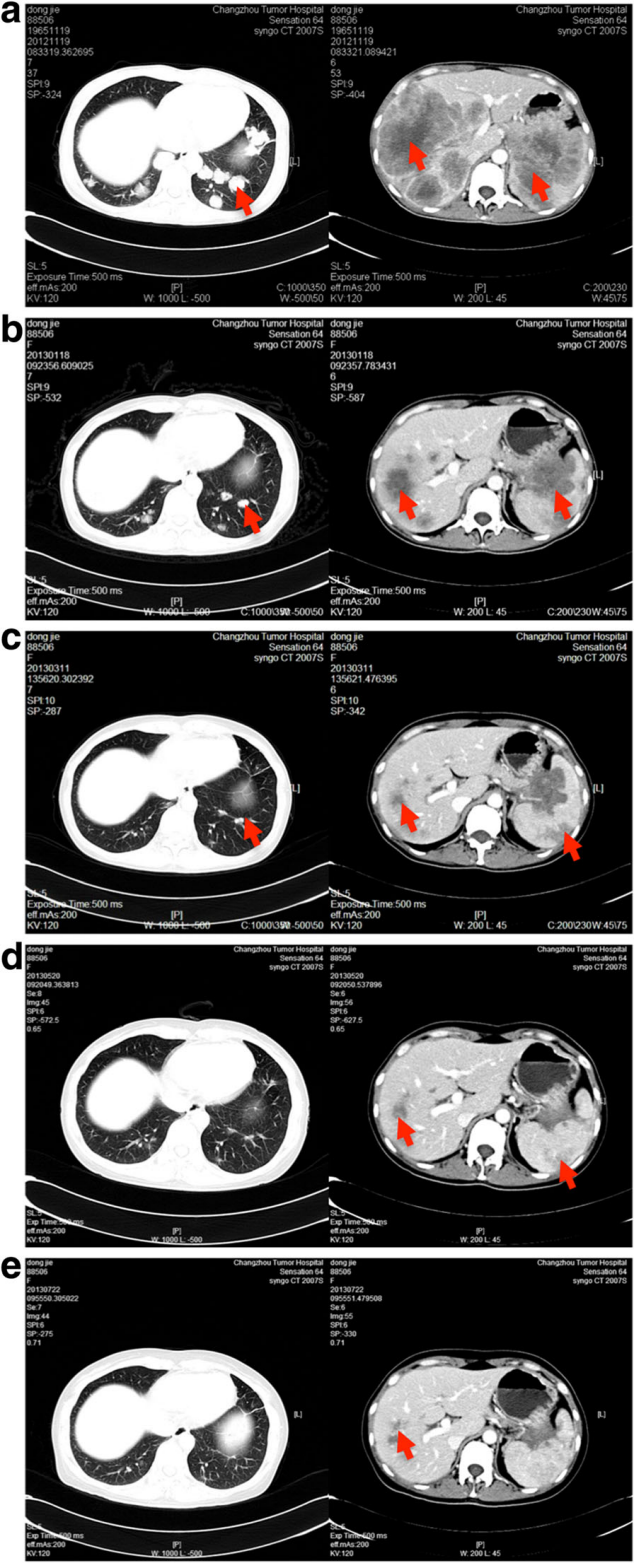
CT results showing the metastatic lesions in the liver, lung and kidney before (a), four (b), eight (c), and twelve cycles (d) after cetuximab and FOLFIRI rechallenge, and two cycles (e) after cetuximab and capecitabine maintenance therapy. Arrows indicate the lesions
